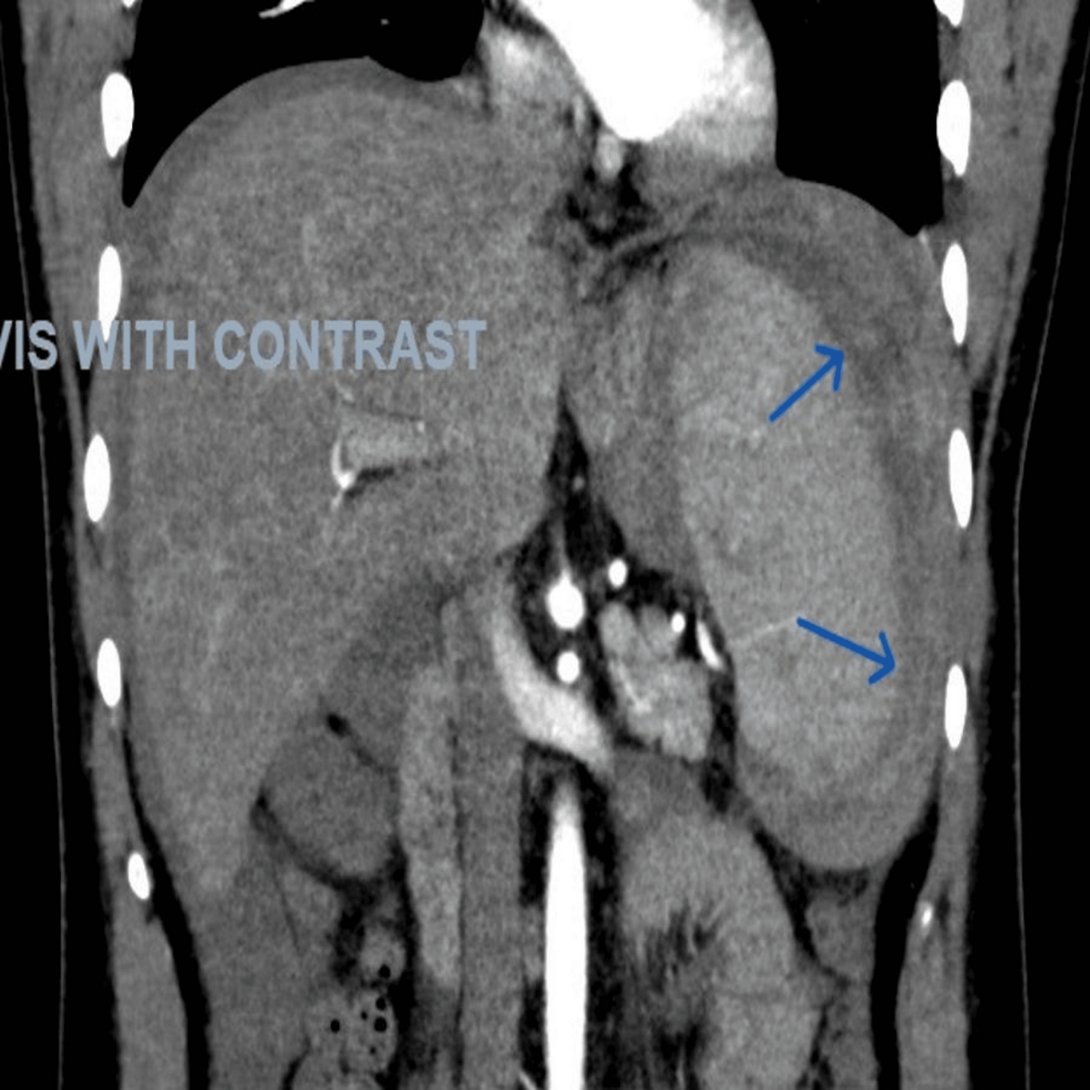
CT angiogram of the abdomen in the sagittal plane showing a peri-splenic collection (blue arrows)
